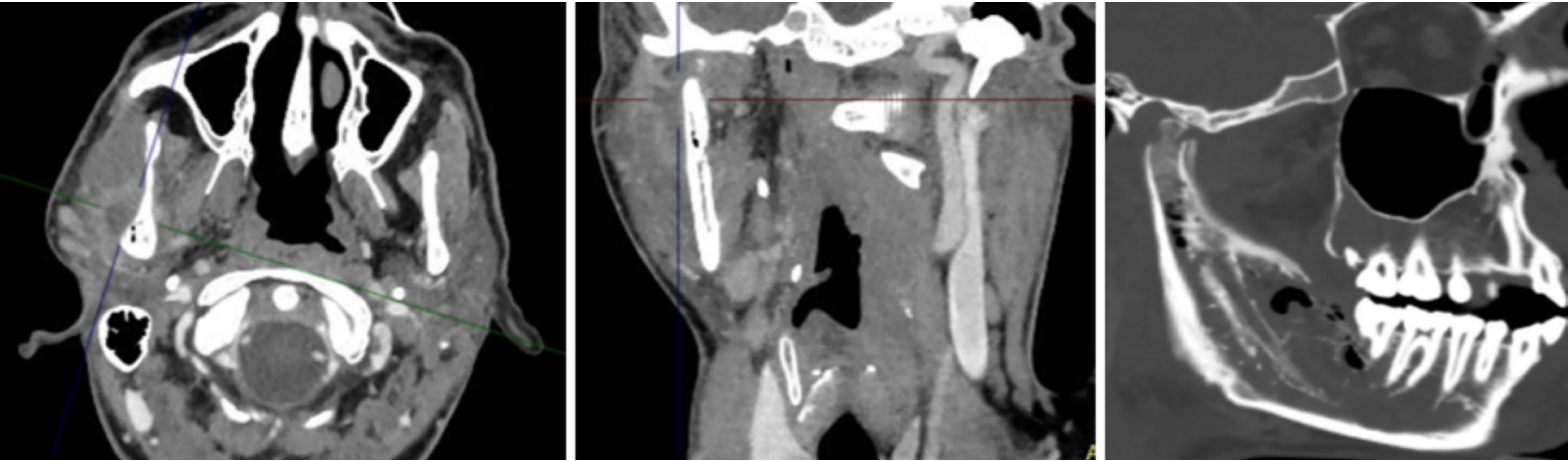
Contrast-enhanced CT scan.
